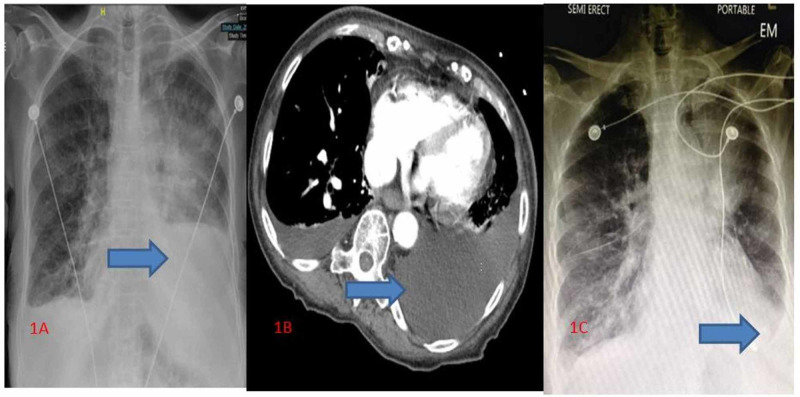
Chest X-ray, chest CT, and chest X-ray post-thoracentesis (A) Chest X-ray showed large left pleural effusion, minimal right pleural effusion, and left upper lobe infiltrate. (B) CT scan of the chest showed large left pleural effusion with minimal right pleural effusion. (C): Chest X-ray post-thoracentesis showing decrease in pleural effusion
